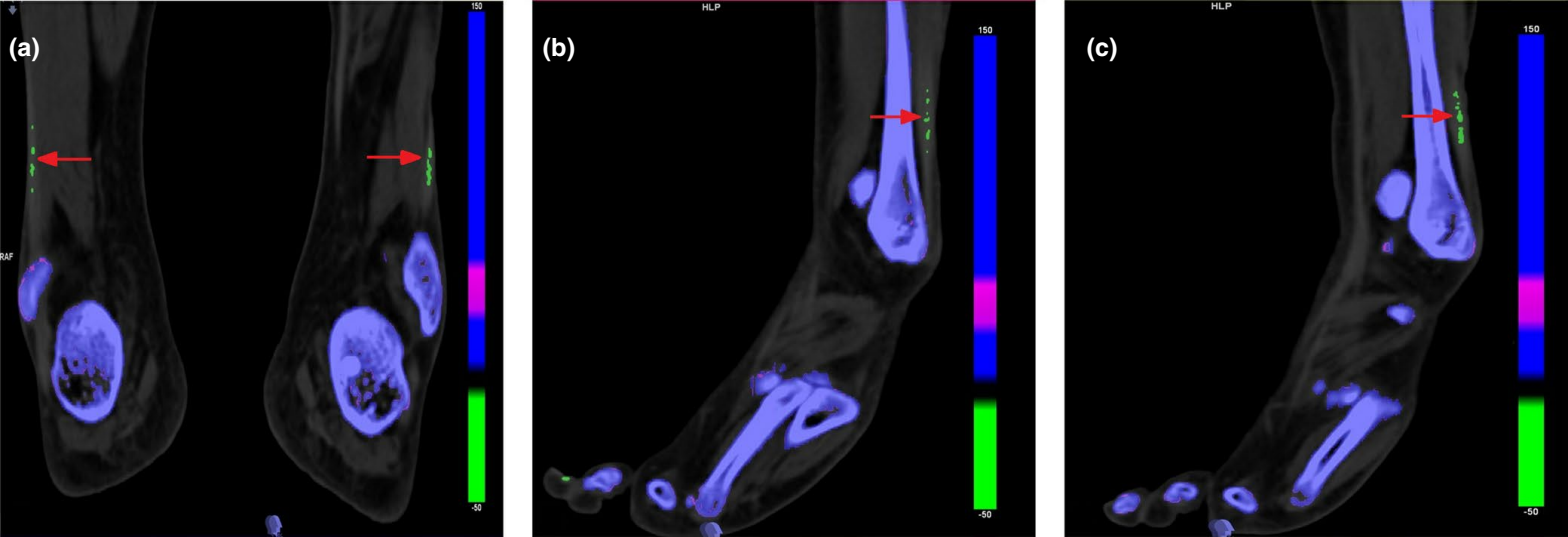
The Biped dual‐energy CT post‐processing results for patient 9. The red arrow indicates gout nodules. a: Bilateral; b: Right; c: Left
